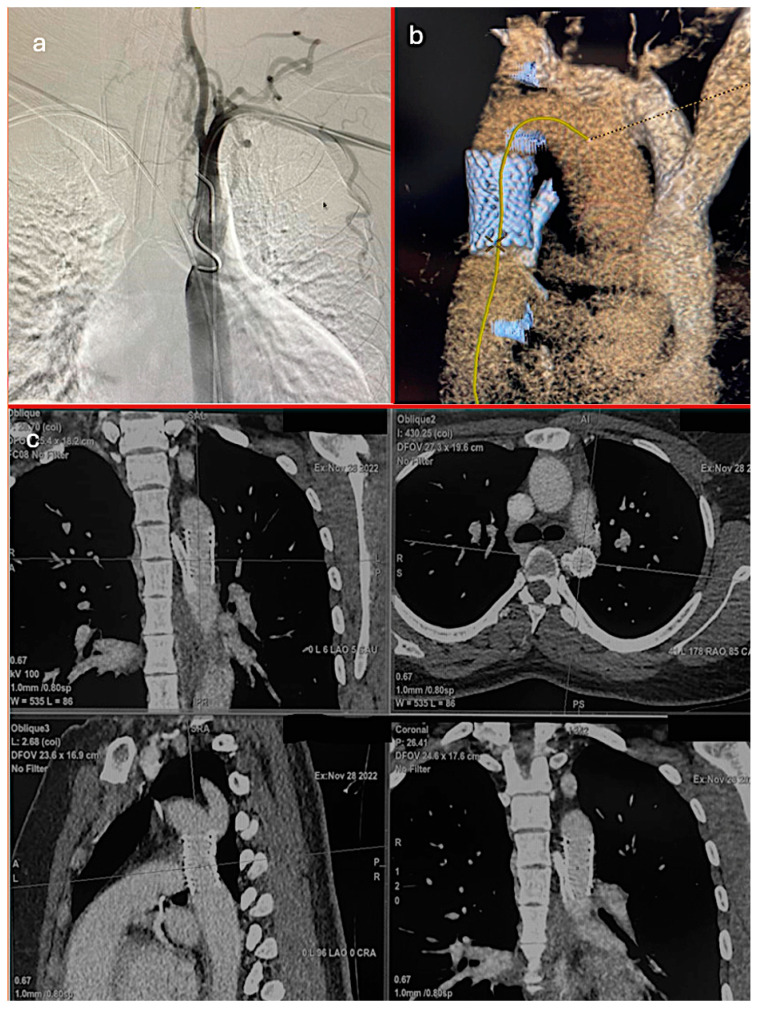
(a) Preoperative angiography of coarctation, (b) postoperative 3D CT imaging of aortic graft and chimney ARSA stent, (c) postoperative CT imaging of aortic graft and chimney ARSA stent.
